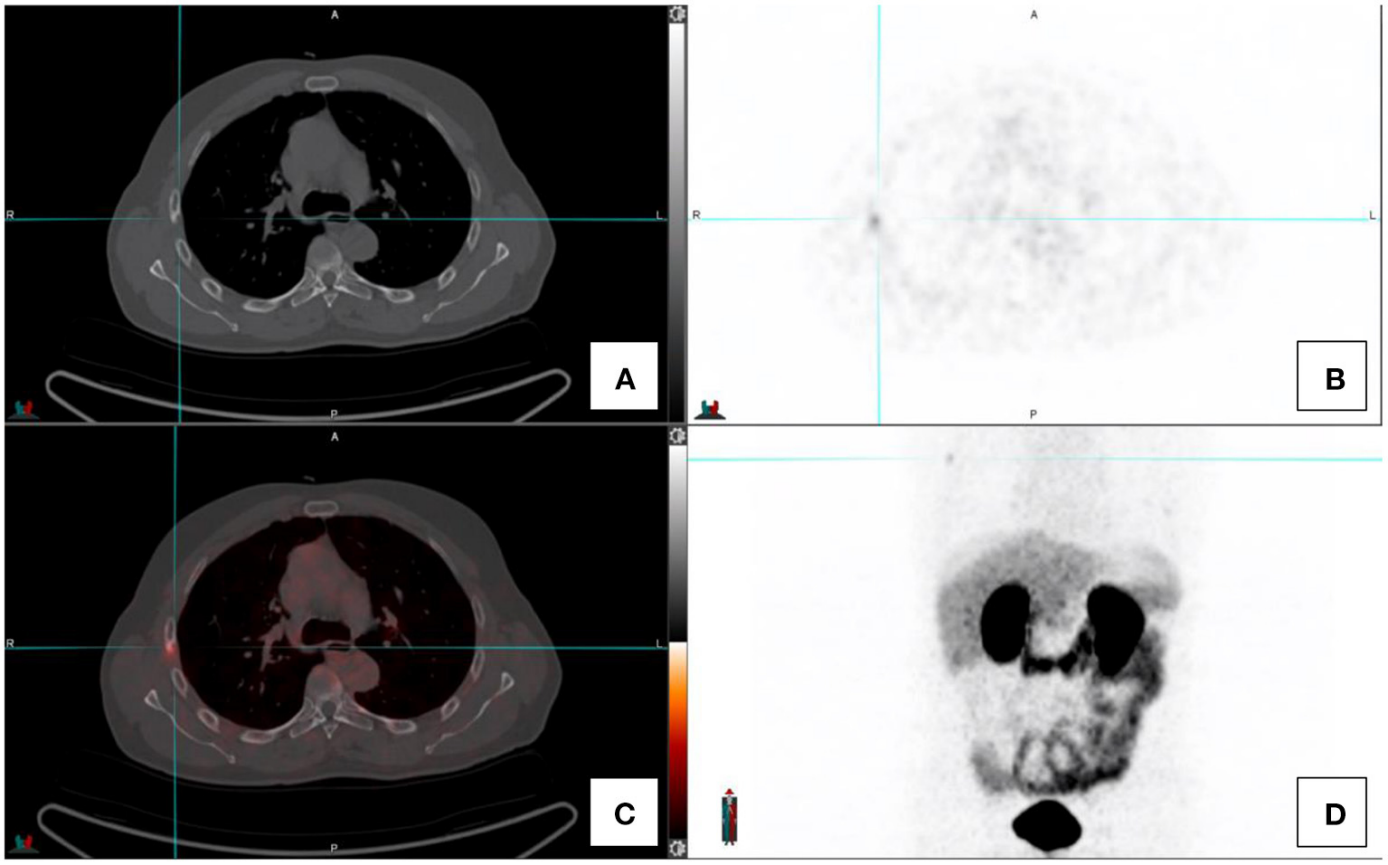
Transaxial images in a prostate cancer patient with a right sided rib fracture. CT-image (A), PET image (B), fused PET/CT image (C), and the maximal intensity projection image (D).
